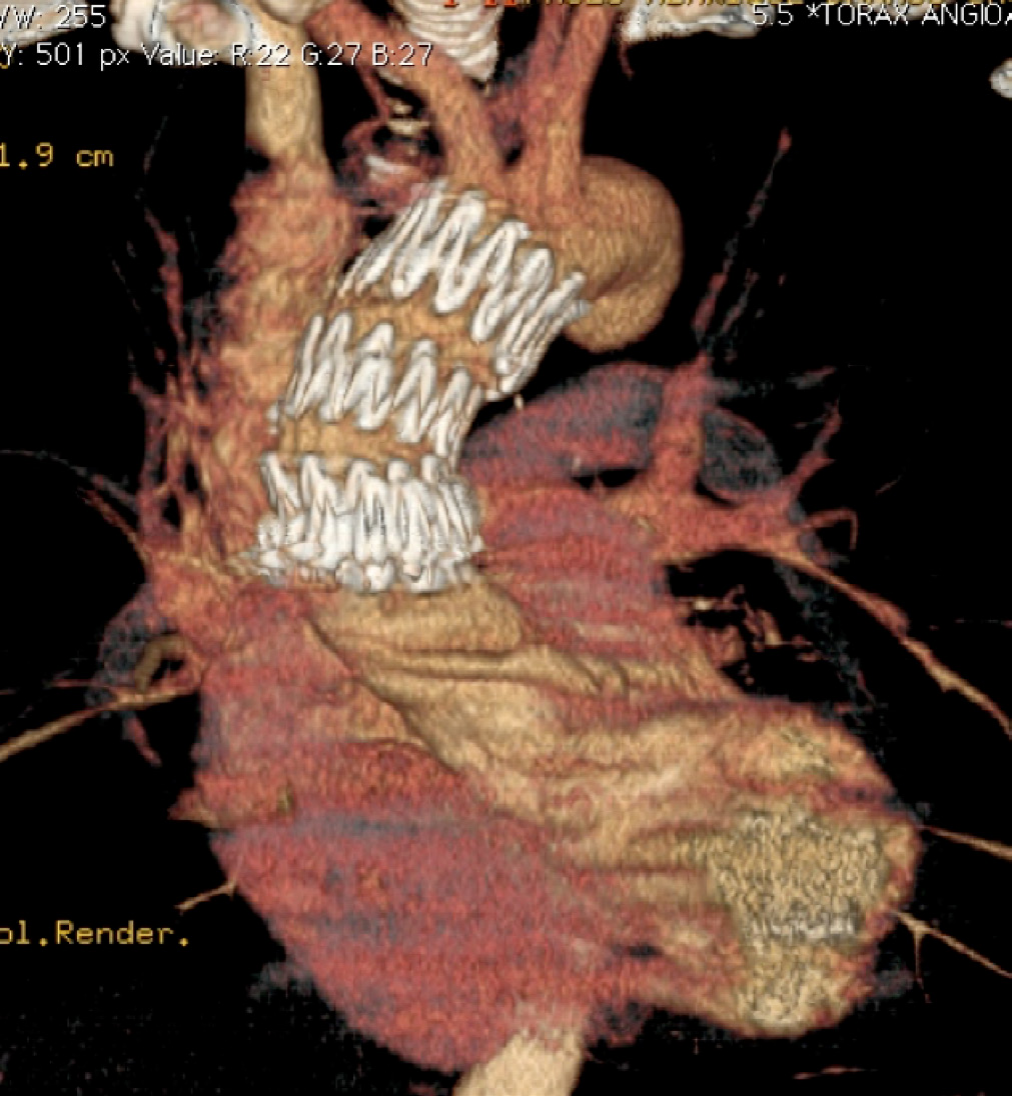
Three-dimensional computed tomography post stent graft in type A aortic dissection.
